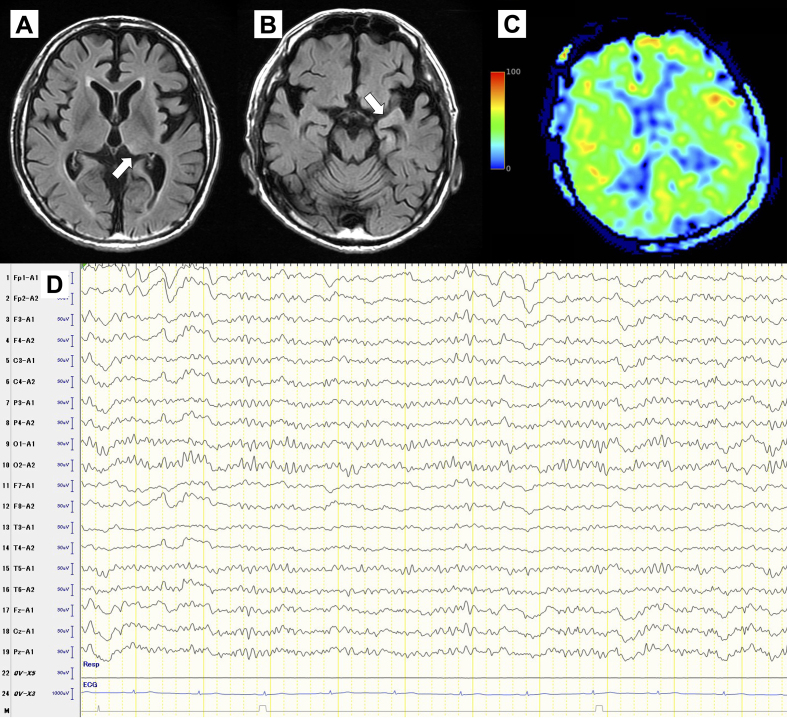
(A) and (B) Fluid-attenuated inversion recovery (FLAIR) on day 8, showing resolving hyperintensity in the left pulvinar (A) and temporal tip (B). (C) Arterial spin labeling (ASL) on day 8, demonstrated no increased signal intensity. (D) Electroencephalography (EEG) on Day 50, showed resolved lateralized periodic discharges.
